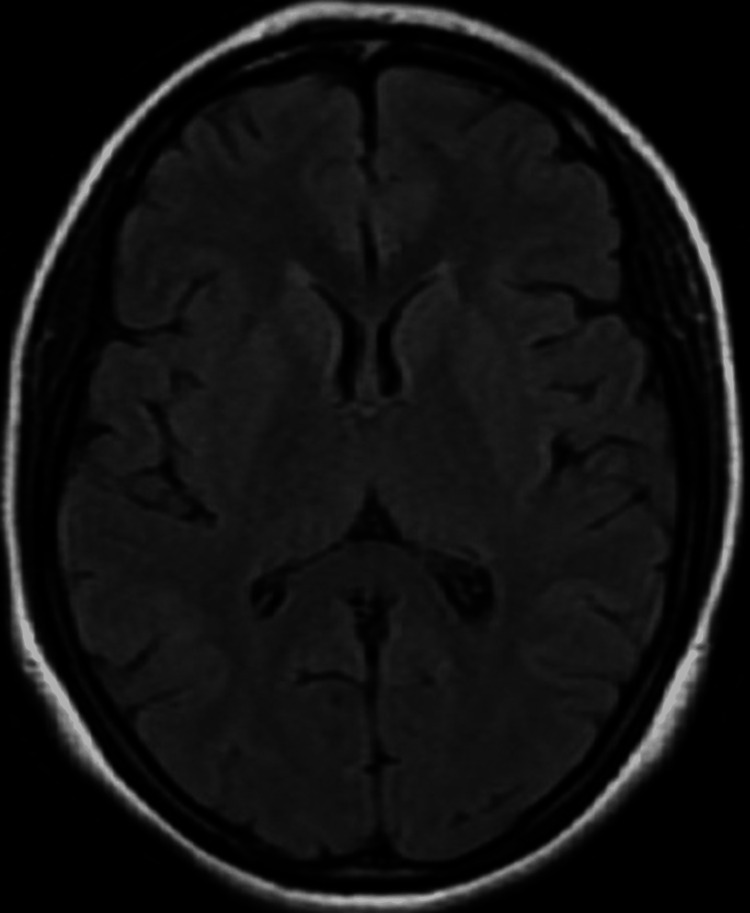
MRI of brain in T2 FLAIR: normal
